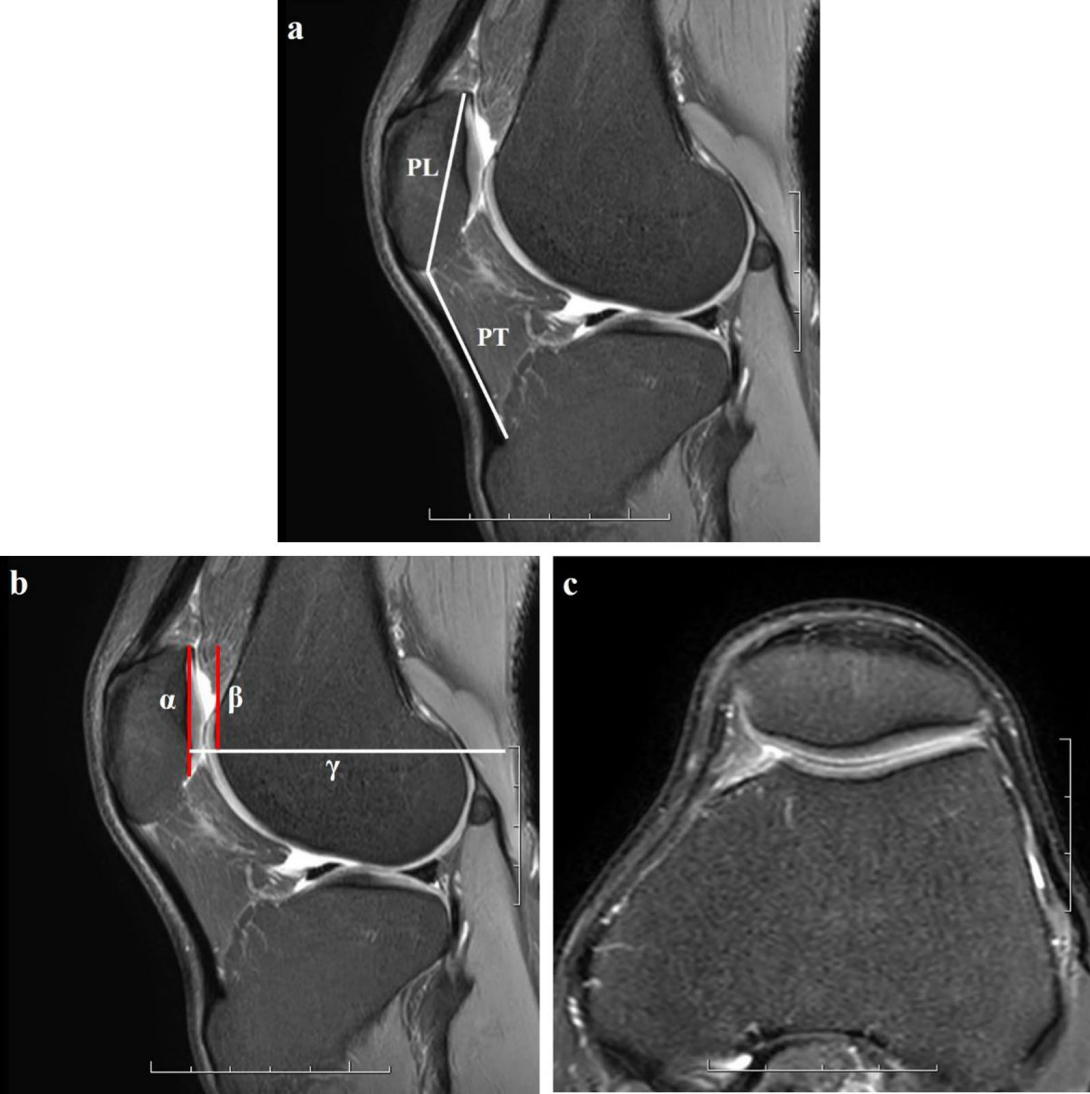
Methods for determining the Insall–Salvati ratio (a) and SP-ET index (b), demonstration of measurements of the related parameters on sagittal MR images. a The Insall–Salvati ratio was calculated as the patellar tendon length (PT) divided by the maximum patellar diagonal length (PL); b the SP-ET index was calculated as the distance between the most superior point of patella and the entrance of femoral trochlea (*β*) divided by the patellar articular surface length (*α*). Line *γ* was the reference line of the entrance plane of femoral trochlea; c the entrance plane of femoral trochlea, and it was the first craniocaudal plane where the complete cartilaginous trochlea can be seen
